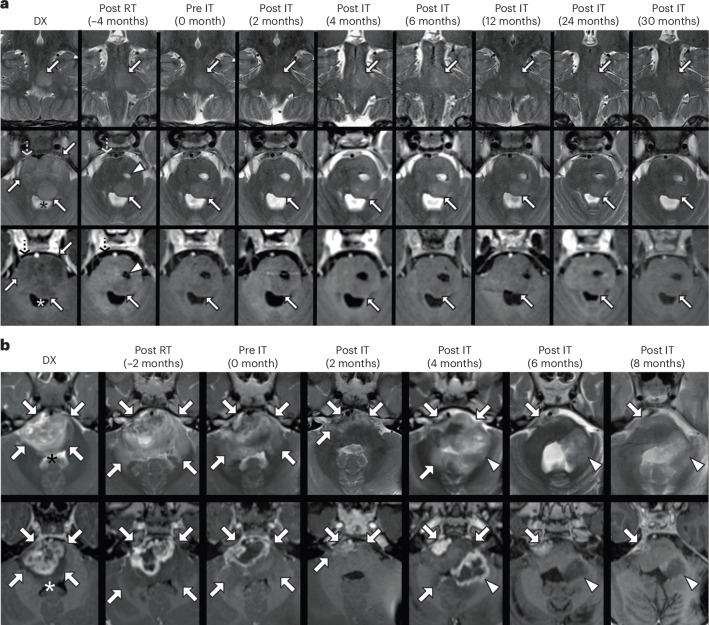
Neuroimaging after locoregional B7-H3 CAR T cell infusion. a, Longitudinal MRIs of S021. T2-weighted (coronal top row, axial middle row) and axial post-contrast T1-weighted (bottom row) MRI images focused on the pontine lesion at various timepoints (see column labels). At diagnosis (DX), the pons is expanded with non-enhancing T2 hyperintensity, prepontine cistern effacement (dashed arrows) and partial effacement of the fourth ventricle (asterisks). Following radiation (Post RT, 4 months before immunotherapy (IT)), the lesion is smaller, with reduced prepontine cistern effacement (dashed arrows), persistent nodular T2 hyperintensity in the left dorsal pons (solid arrows) and biopsy- and therapy-related changes in the central pons (arrowheads). From the initiation of IT (Pre IT) to 30 months Post IT, the overall pons size has remained stable while hyperintensity has decreased. b, Longitudinal MRI of the pontine lesion of S053. Axial T2-weighted (top row) and axial post-contrast T1-weighted (bottom row) MRI images, focused on the pontine lesion at various timepoints (see column labels). At DX, the pons is enlarged (arrows) with diffuse T2 hyperintensity, heterogeneous enhancement and partial effacement of the fourth ventricle (asterisks). Following radiation (Post RT, 2 months before IT), there is further expansion of the pons and increased T2 signal abnormality (arrows), with persistent enhancement. From Pre IT to 2 months Post IT, the pontine lesion is smaller (arrows). However, by 4 months Post IT, the lesion is larger, with a new region of enhancement (arrowheads). At 6 and 8 months Post IT, the lesion size, T2 signal abnormality and enhancement are reduced.
